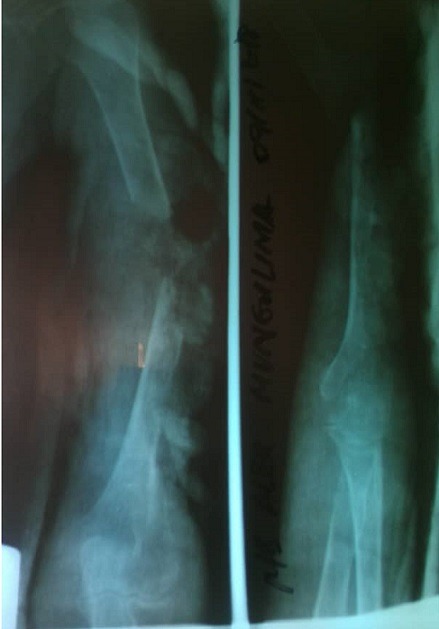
Radiographie montrant une opacité dans les parties molles avec invasion osseuse se traduisant par une perte de substance osseuse, une irrégularité des corticales
